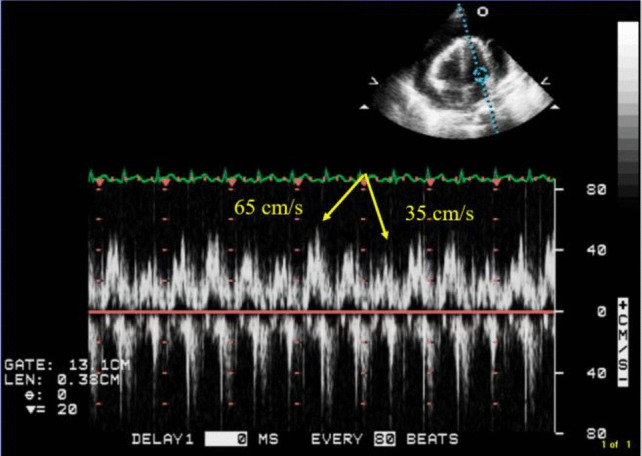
Transthoracic echocardiogram in an apical four-chamber view with doppler at mitral valve demonstrating variation in mitral inflow velocities with respiration
